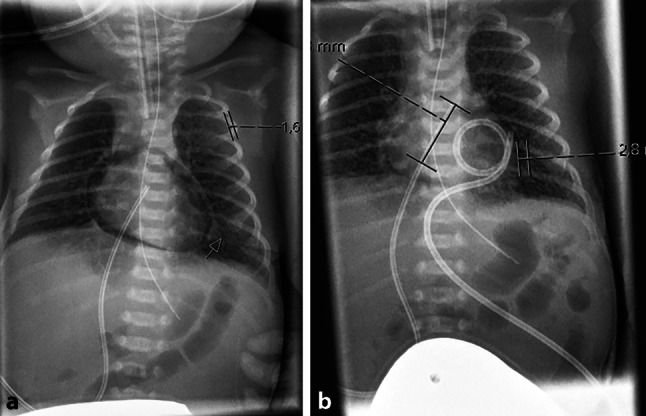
a Chest X-ray demonstrating circular pneumopericardium, b Chest X-ray after insertion of pericardial tube (pigtail)
